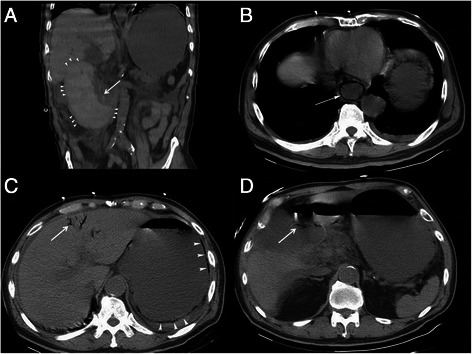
Abdomen and pelvis computed tomography showed a 14.4 cm × 7 cm mass lesion (a, arrowhead) at the lateral side of duodenal second portion and caused lumen narrowing (a, arrow). The distended stomach is suggested to have gastric outlet obstruction. Pneumatosis was found at esophagus (b, arrow), stomach (c, arrowhead) and the bulb (d, arrow). The inflated air also entered the portal system (c, arrow)
